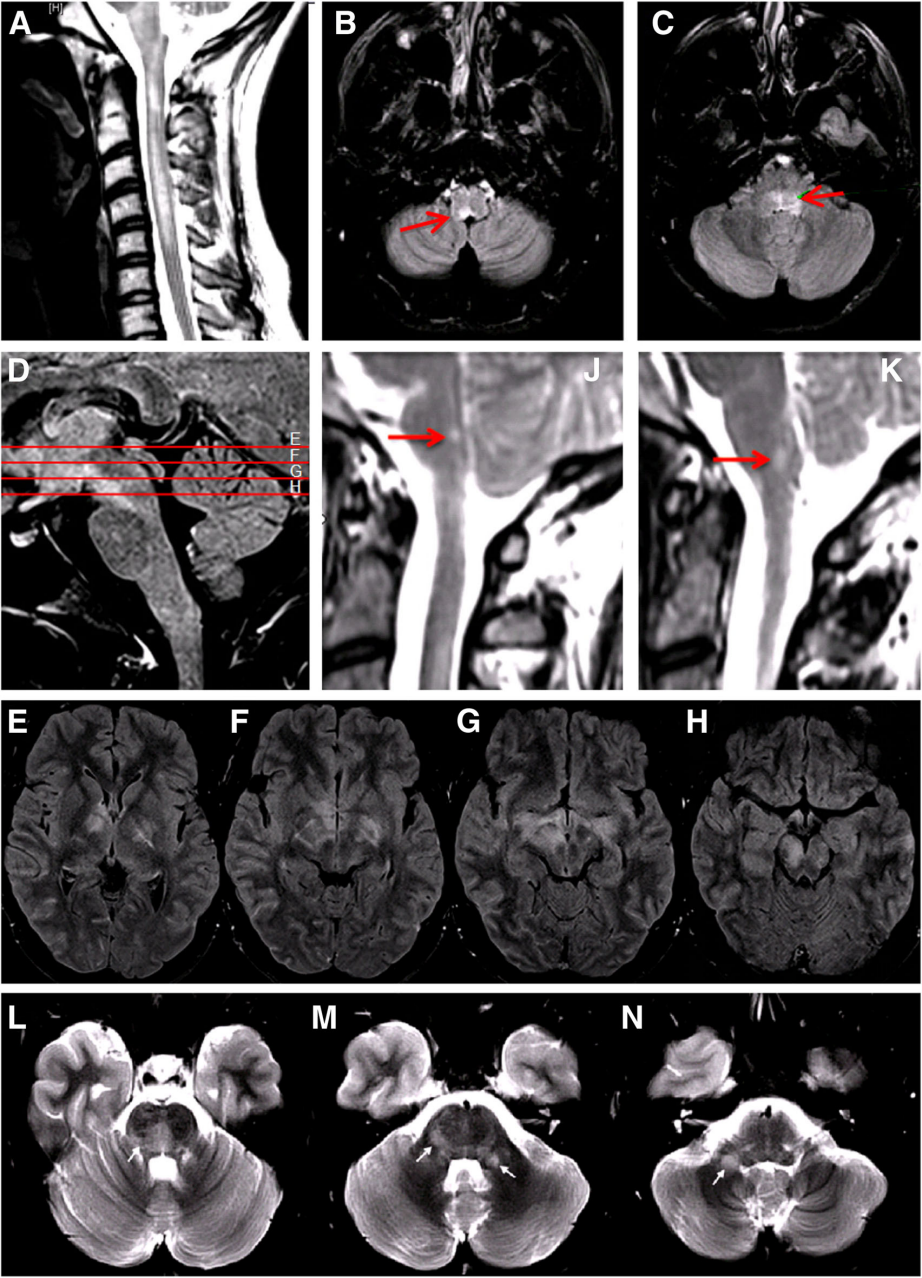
Examples of the magnetic resonance imaging (MRI) findings in patients with MOG-IgG-positive brainstem encephalitis. a-c Patient 12: T2-hyperintense lesions extending from the pontomedullary junction throughout the cervical cord as far as C5; lesions included the dorsal medulla oblongata (B, *arrow*). d-h Patient 8: T2-hyperintense lesions in the pons, midbrain, thalamus, and basal ganglia; lesions involved the periependymal surfaces of the third ventricle. j-k Patient 4: T2-hyperintense lesions in the right (j) and left (k) half of the dorsal medulla oblongata including the area postrema (j). l-n Patient 2: T2-hyperintense lesions in the frontal and parietal subcortical white matter, the pontine tegmentum, and the cerebellar peduncles (*arrows*)
